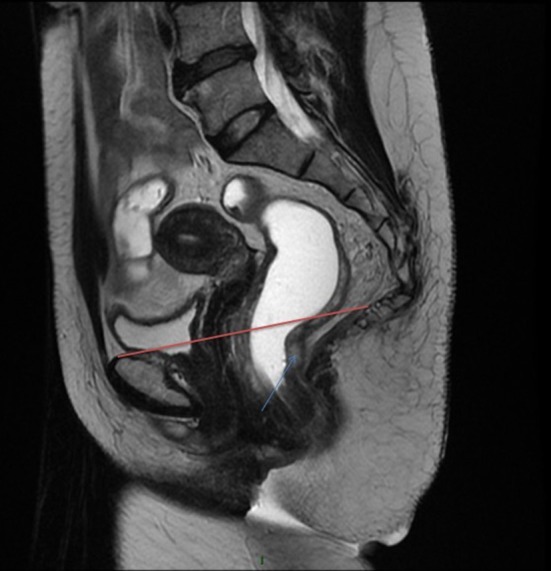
Definition of the low rectum on MRI. On this sagittal view in a MRI T2 sequence, the low rectum begins below the red line the origin of the levator muscles and the pubic bone.
